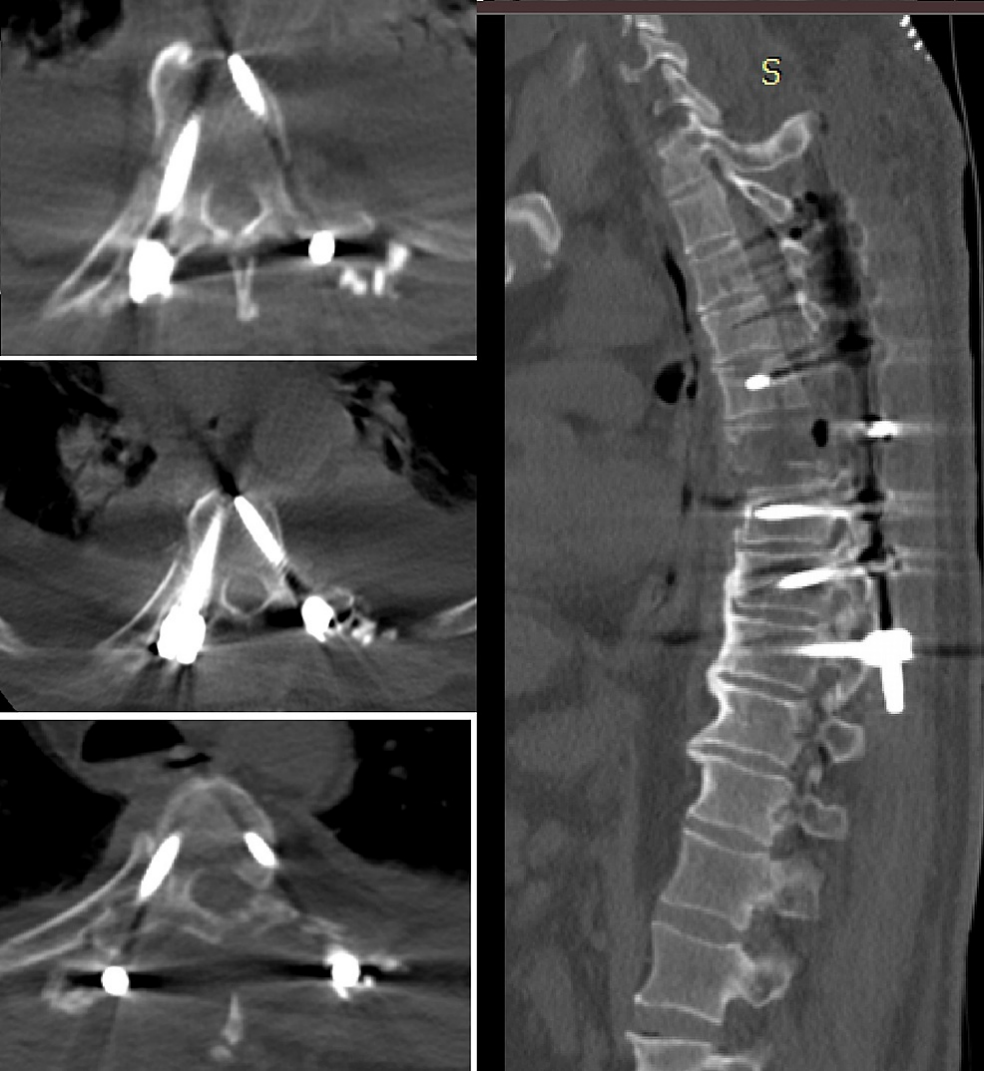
Postoperative CT image of a male patient operated with free hand technique.
